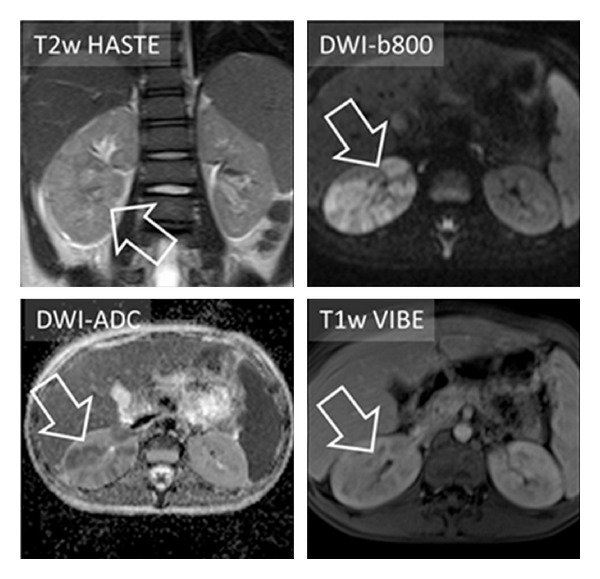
Nine-year-old male patient presenting with pain in the right flank. Laboratory examination revealed increased leukocytes (12.2 × 10^9^ cells/L) and CRP (153 mg/L). MRI scan with routine protocol for the abdomen was performed. It showed only slight signal alterations in T2 HASTE and T1w VIBE (postcontrast). DWI-b800 clearly depicted wedge-shaped, striated areas of high signal intensity in the right kidney with correlating low signal in DWI-ADC. The diagnosis of renal infection was finally done on the basis of clinical criteria.
